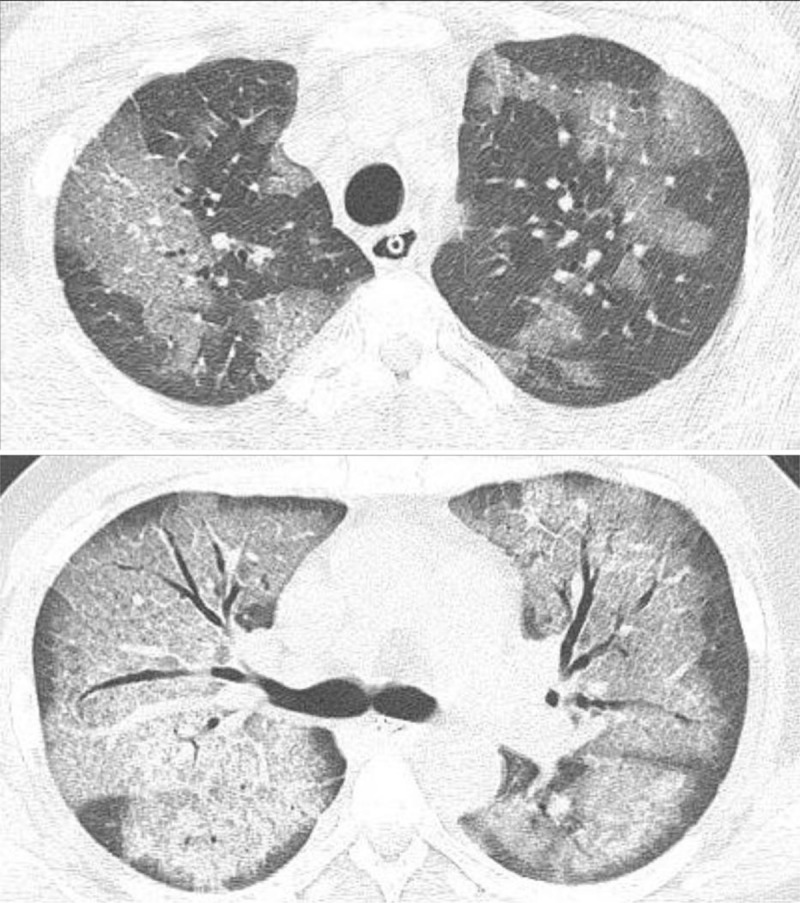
Computed tomography scan performed 28 days after admission, showing diffuse/patchy ground glass opacities and consolidations with interlobular septal thickening in both lungs.
